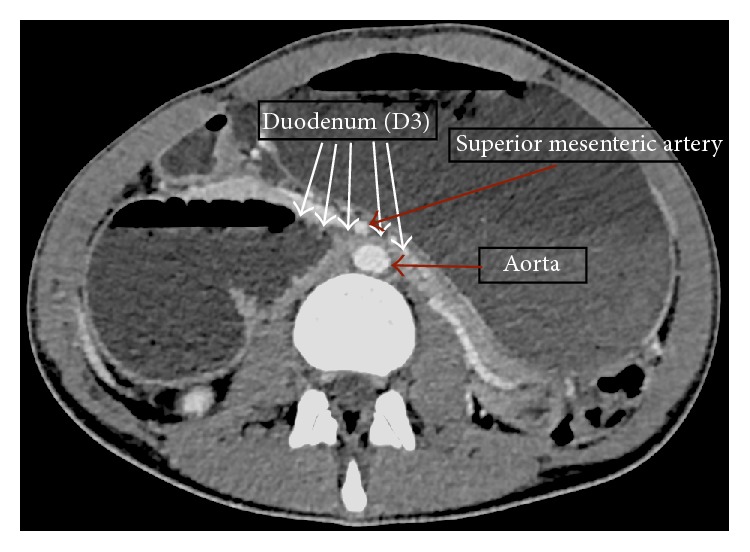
Axial CT section demonstrating the anatomy of SMA syndrome.
